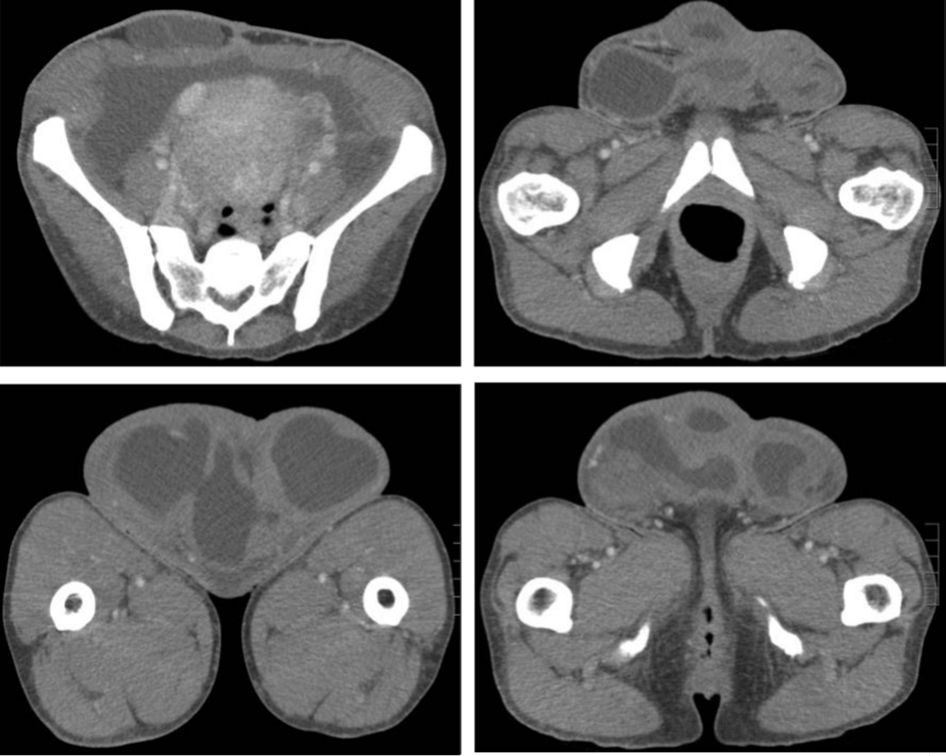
Axial sections from a computed tomography (CT) scan with intravenous contrast that demonstrated a large, extraperitoneal lobulated spaceoccupying lesion with mixed cystic and solid components.
